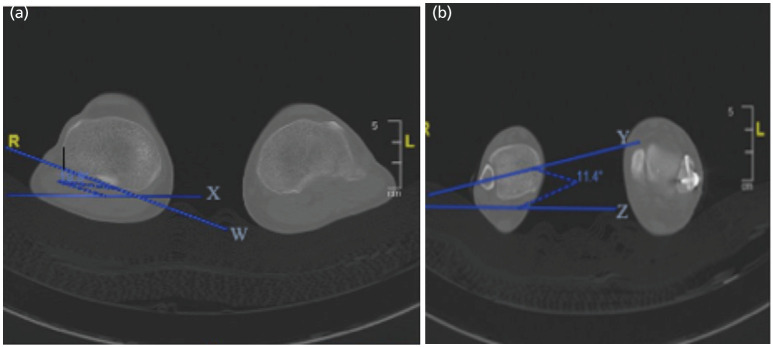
CT Scanogram and technique of measuring rotational profile of the leg. (a) Rotational profile of the leg measured at the knee and (b) above the ankle. (a) A line (line “W”) is drawn tangent to both posterior condyles of the tibial plateau on the CT image just proximal to the fibular head. This angle is measured against the horizontal, labelled line “X”. (b) A distal reference line (line "Y") is drawn through the distal tibia that passes through the centre of the fibula and tibia on a slice just above the distal tibial plafond. This angle is measured against the horizontal, labelled line “Z”. The difference in angles was used to calculate the degree of tibiofibular torsion. The extent of malrotation was assessed as the difference in values between the injured and uninjured limb.
